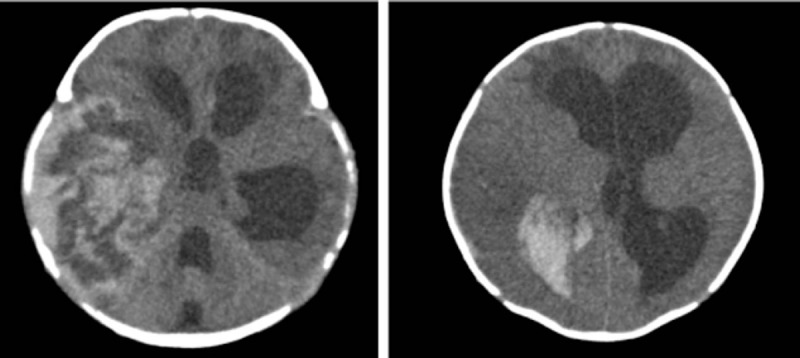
Head computed tomography scans of a female neonate hospitalized for weak cry and poor mental response (case 2). Multiple hematomas are visible in the right iliac occipital lobe and bilateral frontal lobes. The midline appears shifted slightly to the left. Hemorrhage is noted in the right lateral ventricle horns and body, with supratentorial hydrocephalus.
